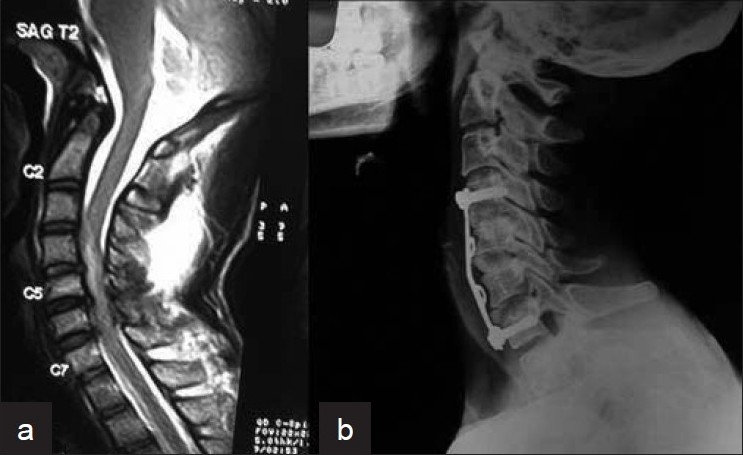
(a) Sagittal MRI T2WI of cervical spine in a posttraumatic cervical spine injury showing three level disc herniation. (b) 16 months post-operative X-ray cervical spine (lateral view) after excision, fusion and fixation showing good bony fusion
